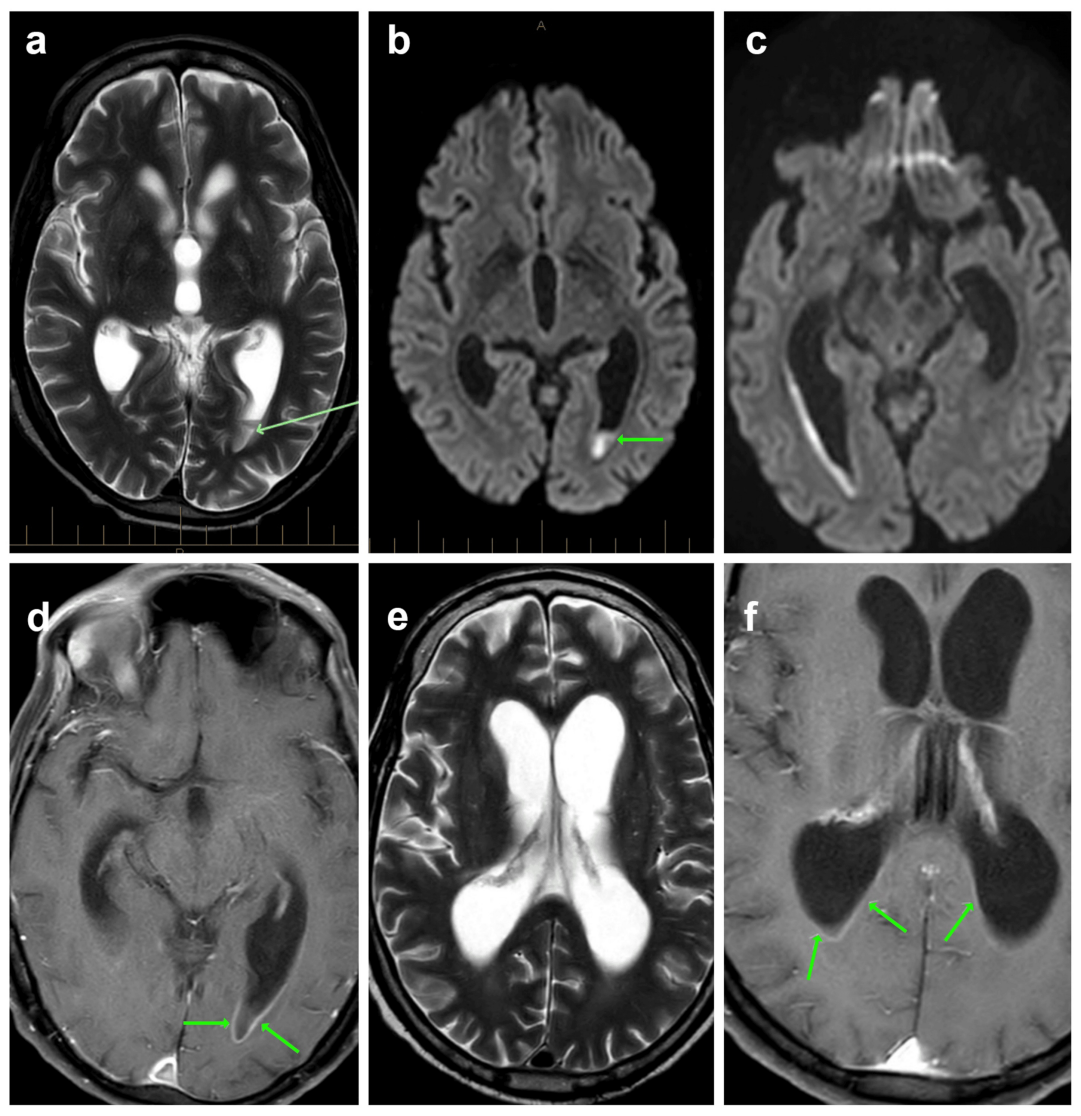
Pyogenic ventriculitis on MRI (a, b) Pus in ventricles in a non-enhanced sequence; (c) diffusion-weighted imaging; (d, f) T1 sequence, post-contrast; (e) T2 sequence demonstrating dilatation of the ventricular system with the smooth ependymal enhancement of the posterior and occipital horns of the lateral ventricles indicating ventriculitis.
